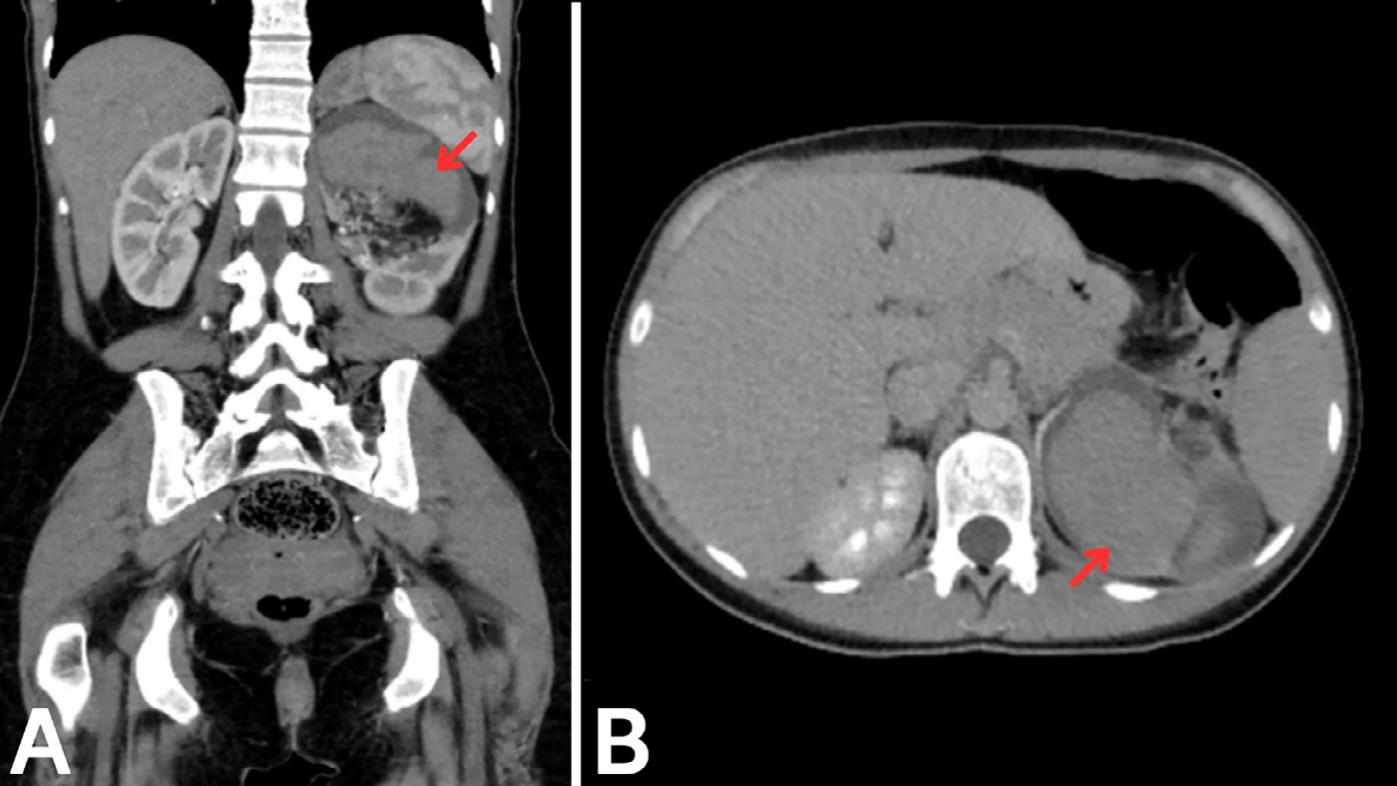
Follow-up CT with contrast showing ruptured AML. A: Coronal plane in the portal phase showing a newly developed hyperdense hematoma (red arrow) within the left renal mass, consistent with rupture. B: Axial plane in the portal phase confirming the presence of the hematoma (red arrow) and its localization within the mass.
